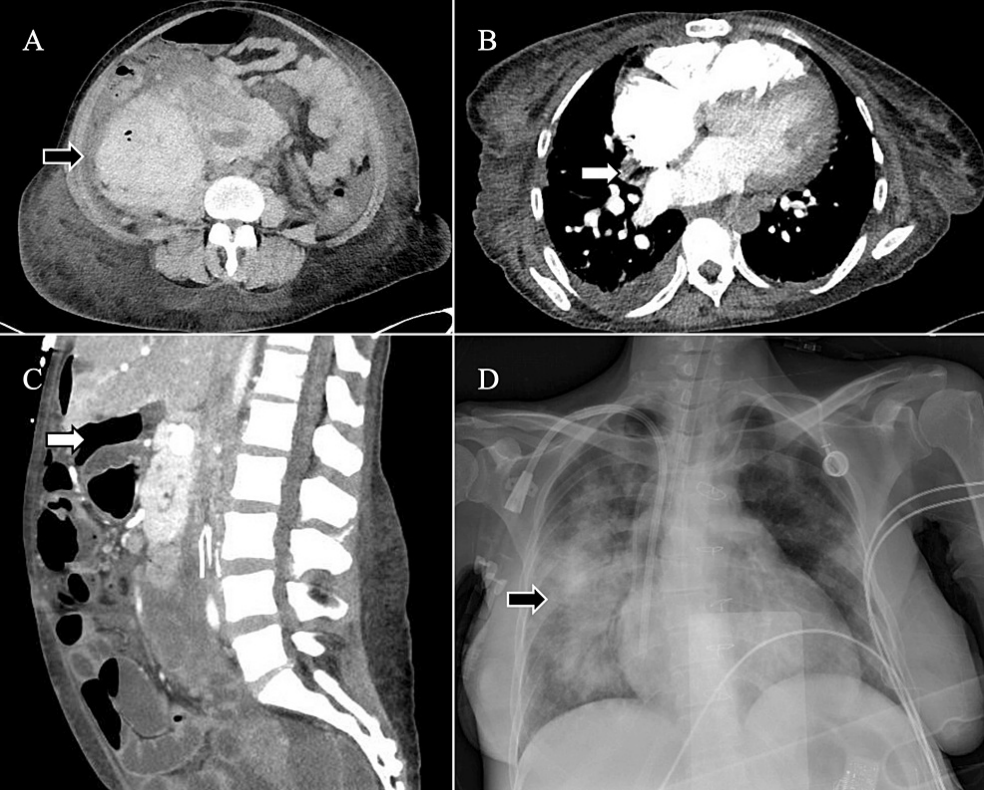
Imaging findings of complications during the patient's hospital course. A: Right perinephric hematoma (black arrow) measuring 15.8 x 10.7 cm; B: Filling defect within the subsegmental branch of the right lower lobe pulmonary artery (white arrow); C: Sagittal image showing pneumoperitoneum (white arrow); D: Chest radiography (CXR) showing right perihilar opacities (black arrow).
